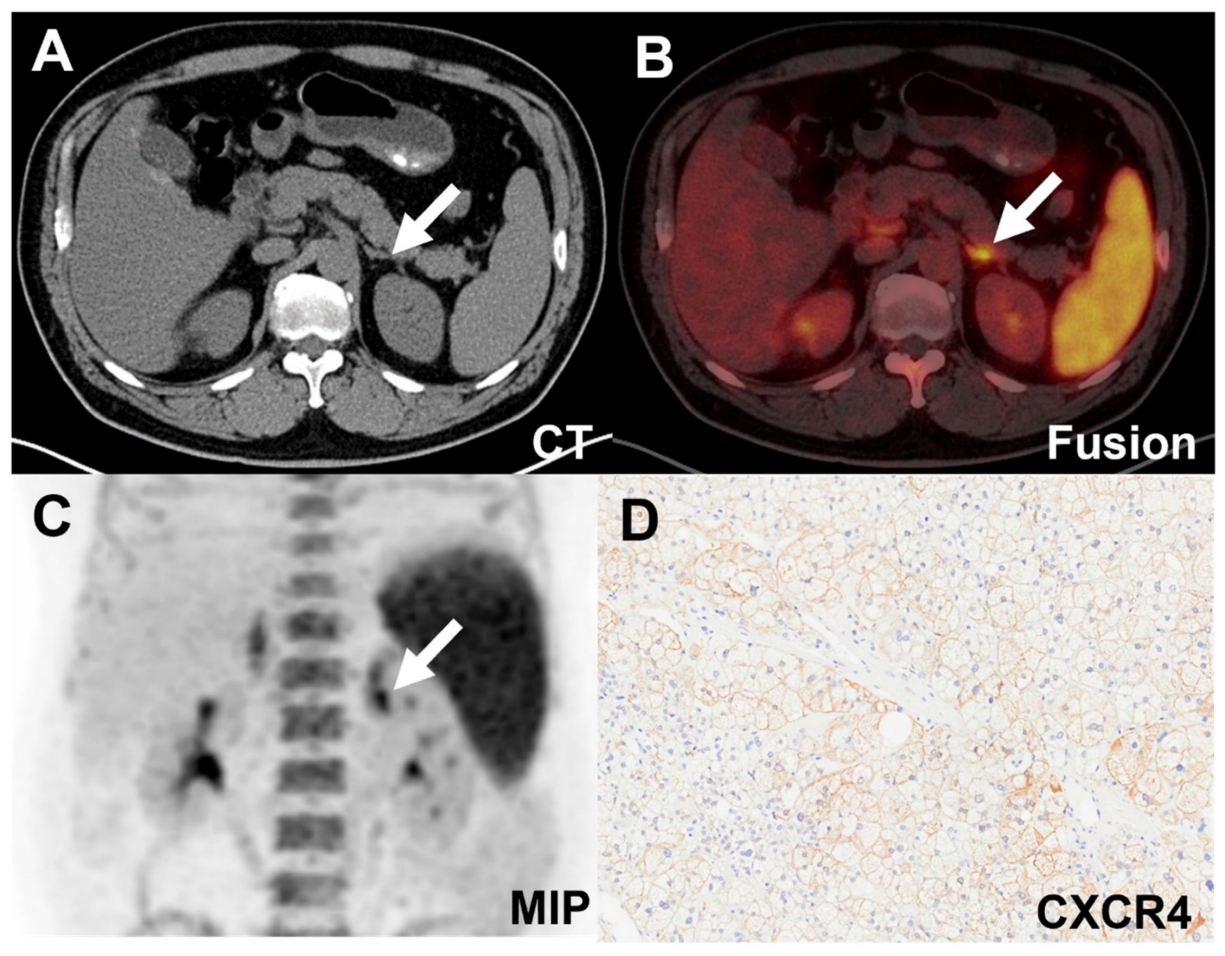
False-positive uptake (SUVmax 11.08, white arrow) in an IHA lesion on [^18^F]AlF-NOTA-pentixather PET/CT scan. A-C. PET/CT showed increased activity in the left adrenal nodule. D. Expression of CXCR4 was demonstrated in the adrenal hyperplastic tissue.
